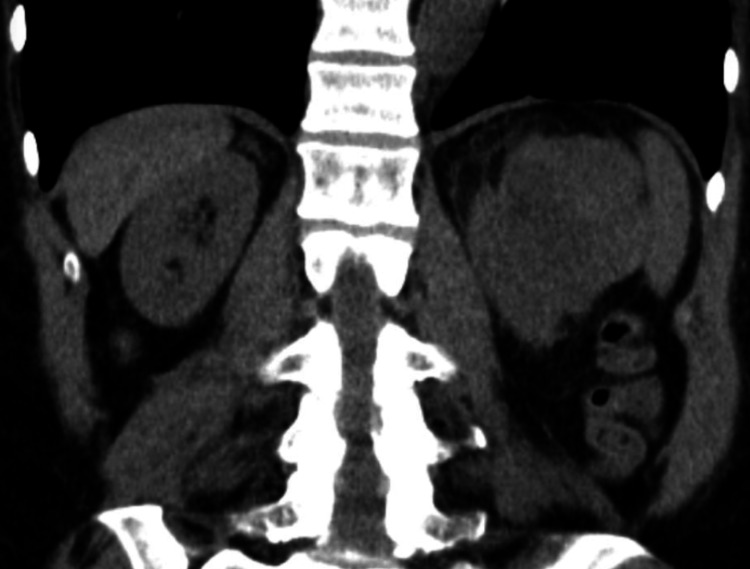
Non-contrast abdominal CT. Lobulated, heterogenous mass arising from the superior posterolateral aspect of the left kidney with focal hyperdense and hypoattenuating areas.
